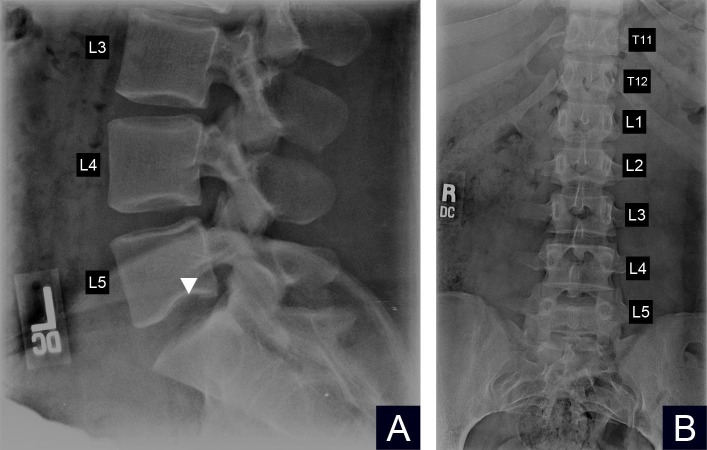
Figure showing the plain radiographs of the lumbar spine A) Lateral view of the lower lumbar spine showing a small Schmorl’s node in the inferior endplate of lumbar spine (L5) (white arrowhead) B) The anteroposterior view of the lumbar spine showing unremarkable findings
